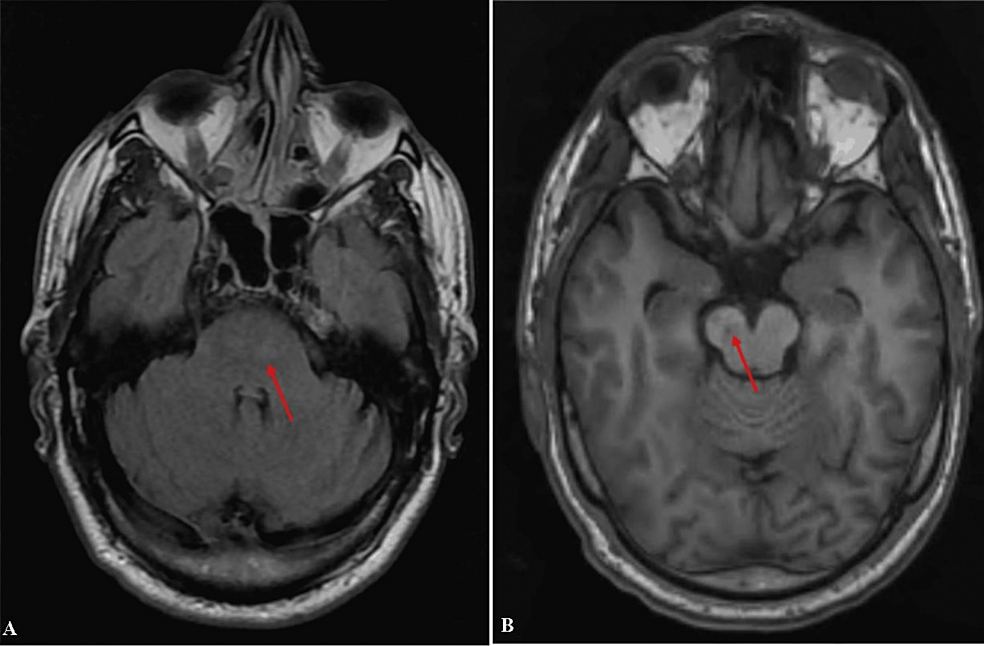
Axial Flair sequence cerebral MRI Cerebral MRI, Axial Flair sequence, showing a flair expression of the ischemic stroke in the pons (A) and the anterior cerebral peduncule (B)
